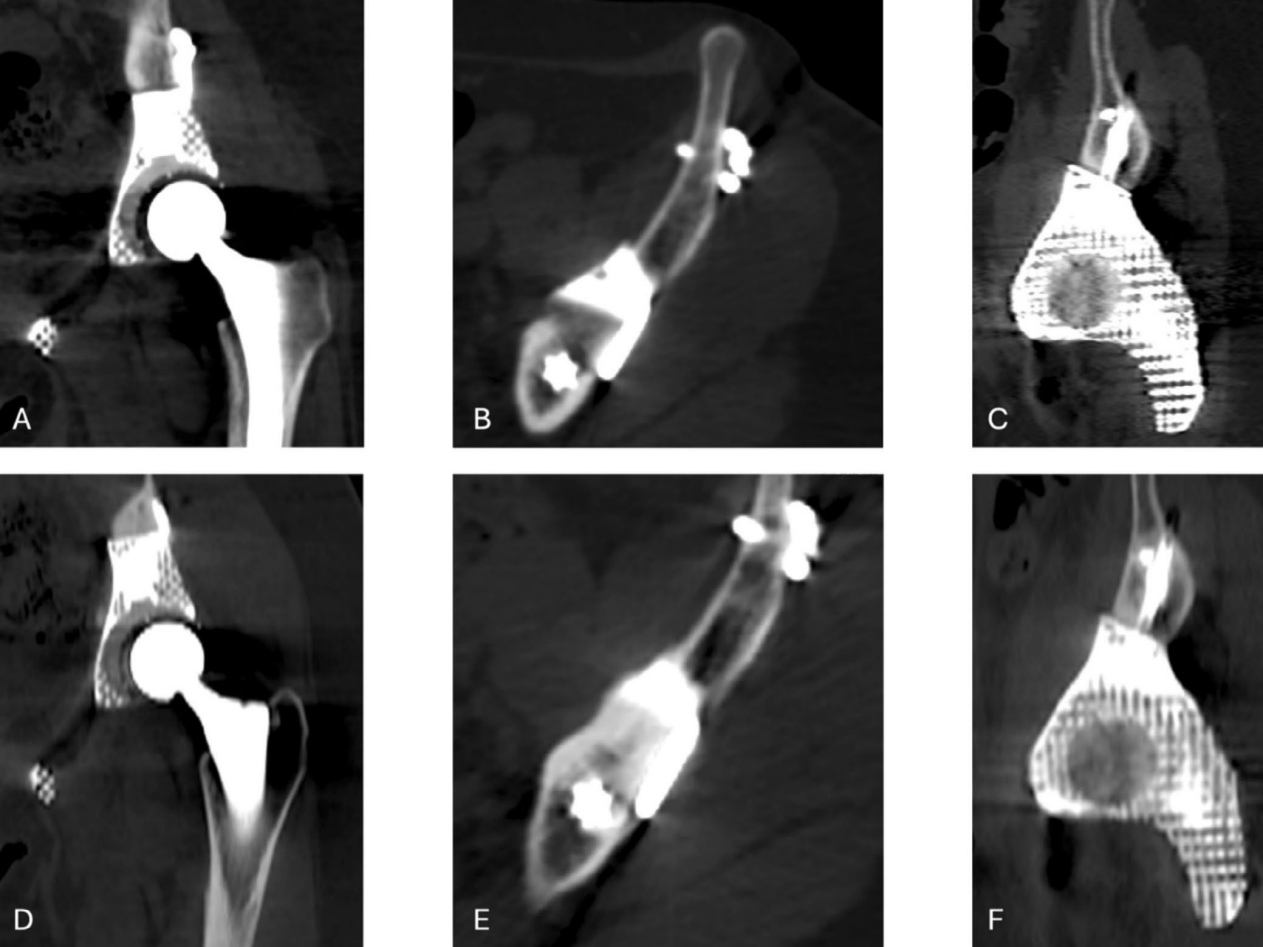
Postoperative CT images in (A) coronal, (B) axial, and (C) sagittal views show the interface between the implant and the host bone. A radiolucent line is visible along the bone–prosthesis contact surface. CT images in (D) coronal, (E) axial, and (F) sagittal projections at 5‐year follow‐up demonstrate the absence of the radiolucent line and the presence of newly formed bone, with clear evidence of bone ingrowth around the implant, indicating stable and long‐term osteointegration.
